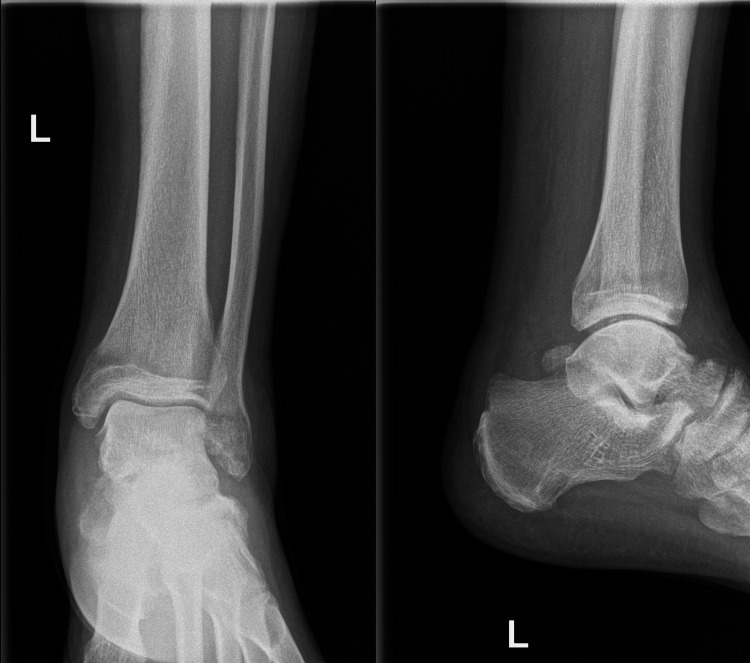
Anteroposterior(AP) and lateral view X-rays of the left ankle at three months
